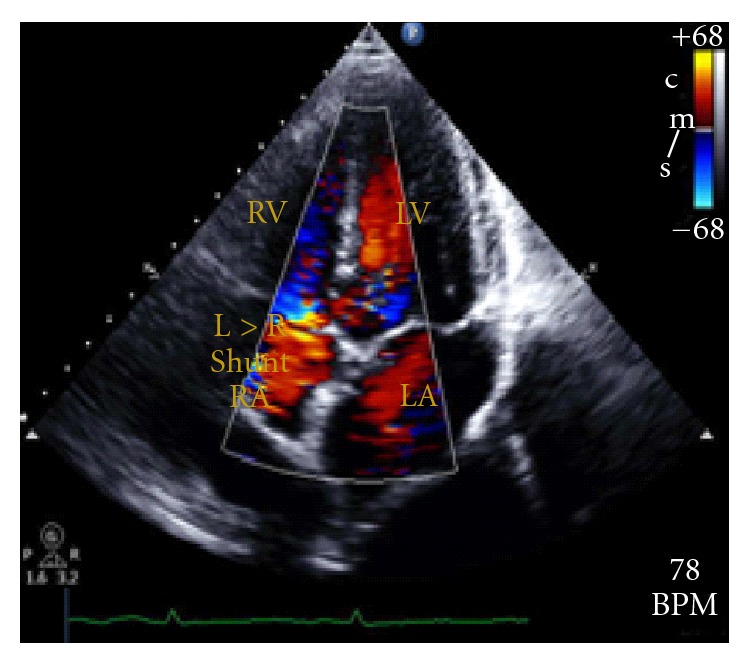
Apical four chamber view showing the presence of a patent foramen ovale with predominant left-to-right shunt.
